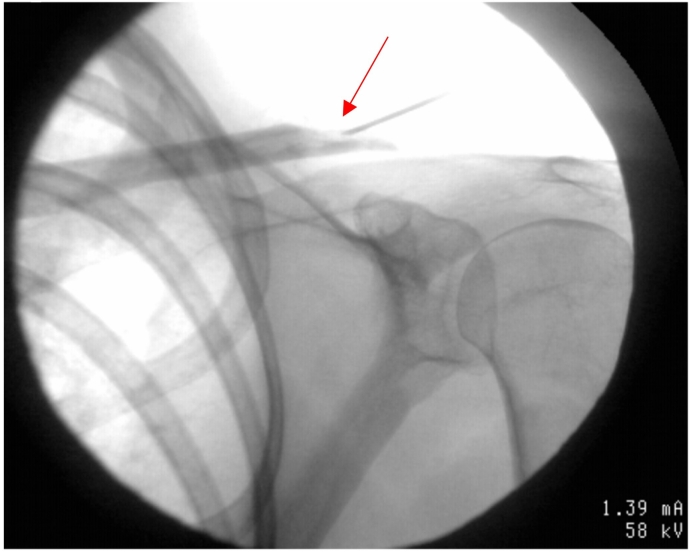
Fluoroscopically assisted needle biopsy (red arrow) after finding of continued distal left clavicle osteolysis after revision surgery, removal of implants, and four week course of intravenous Vancomycin. MRI demonstrated resolution of osteomyelitis. Cultures of the biopsy demonstrated no growth of *C. acnes* three weeks after. (For interpretation of the references to colour in this figure legend, the reader is referred to the web version of this article.)
